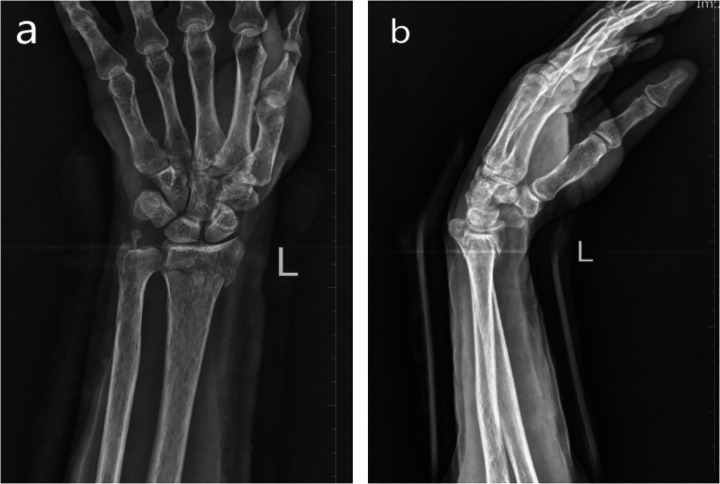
Anteroposterior and lateral wrist DRs at the two-week follow-up. (a,b) mild radial displacement of the distal radius with no abnormalities observed in the DRUJ. DR, digital radiograph; DRUJ, distal radioulnar joint.
